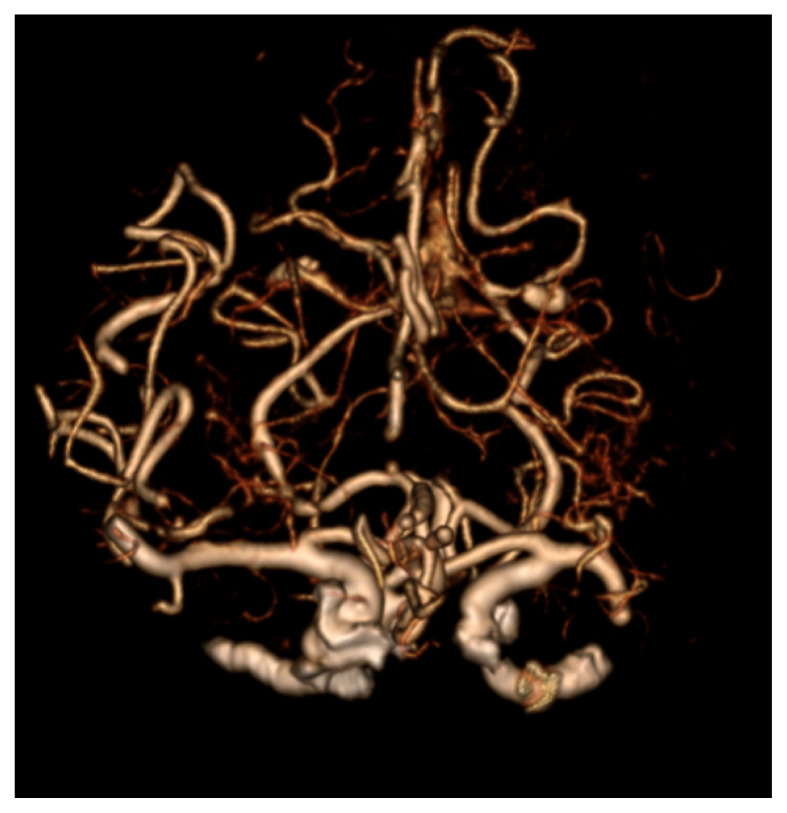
Maximum Intensity Projection image from CT Angiography (CTA) of Madam M demonstrating abrupt tapering at the distal segment of the left M1 branch of the middle cerebral artery, suggestive of a large vessel occlusion
